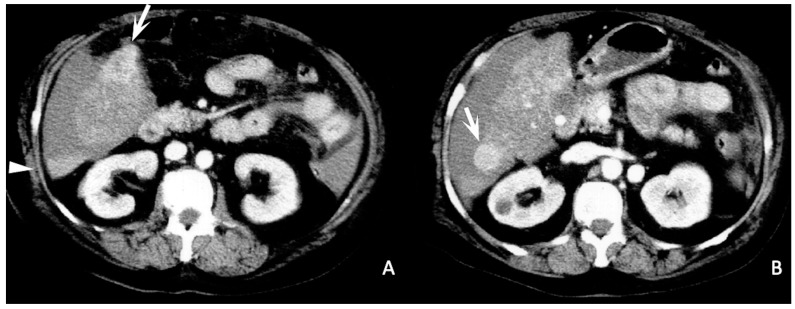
Arterial phase enhanced CT scan. Subcapsular HCCs (arrows (A,B)) surrounded by peri-hepatic hematoma. A double fluid-fluid level with a hyperdense clot in dependent position is clearly visible (the hematocrit sign and the sentinel clot sign) (arrowheads (A)).
